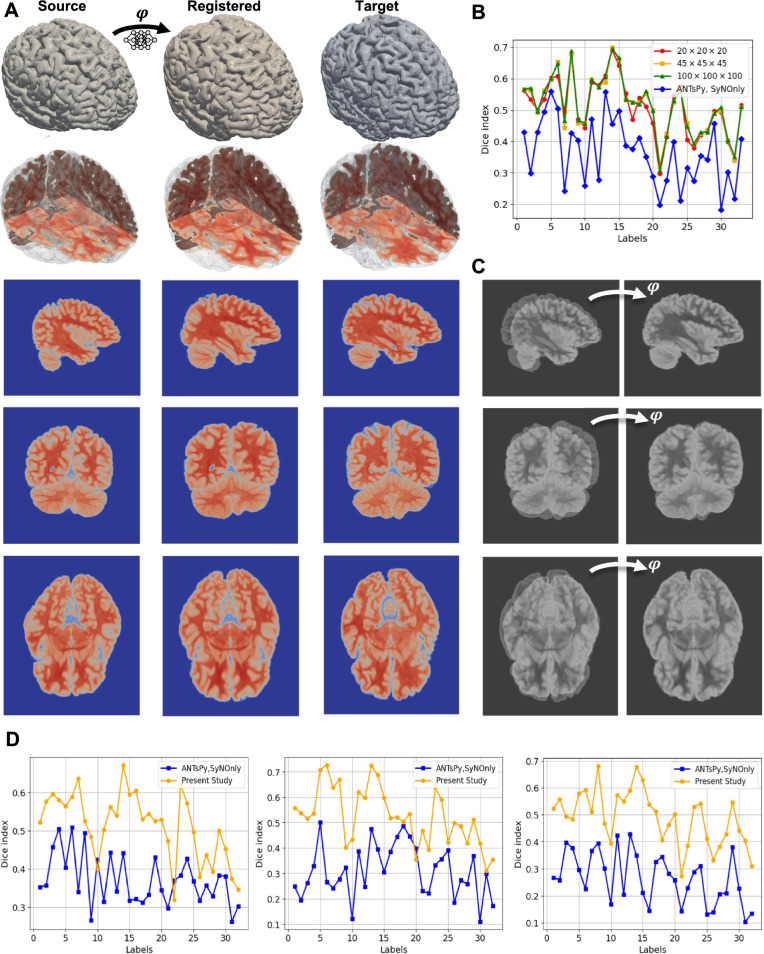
Benchmark problem for brain registration. A) Iso-surfaces along with sample sagittal, coronal, and axial cross sections corresponding to source, registered, and target of NIREP MRI datasets MRIs (na10.nii.gz to na02.nii.gz). B) Comparison of registration methods in terms of the Dice index between the current method across different discretization sizes and ANTsPy (SyNOnly) for all ROIs in the dataset. C) Overlay of initial and target intensities as well as the target and deformed geometry from the registration process, showing good alignment was achieved. D) Comparison of the result of registrations for three more pairs chosen from NIREP data sets(left: na16.nii.gz to na05.nii.gz, middle: na11.nii.gz to na03.nii.gz, and right: na12.nii.gz to na01.nii.gz)
